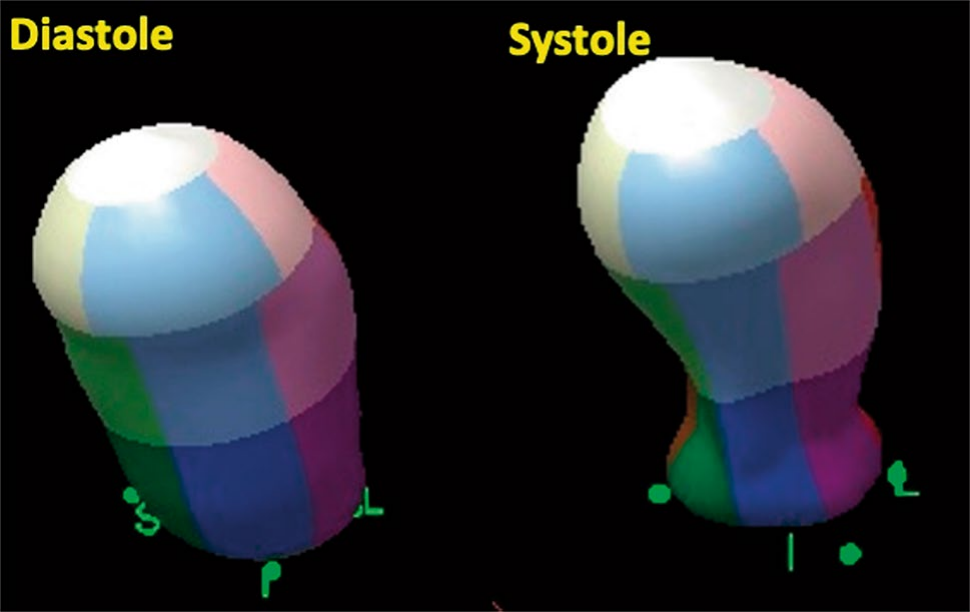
3D assessment of wall motion abnormality. In addition to quantification of volumes, 3D echocardiography is useful for visual confirmation of patterns of wall motion abnormality. The left and right panels indicate an end-diastolic and end-systolic phase, which clearly depicts apical ballooning with basal hyperkinetic motion
